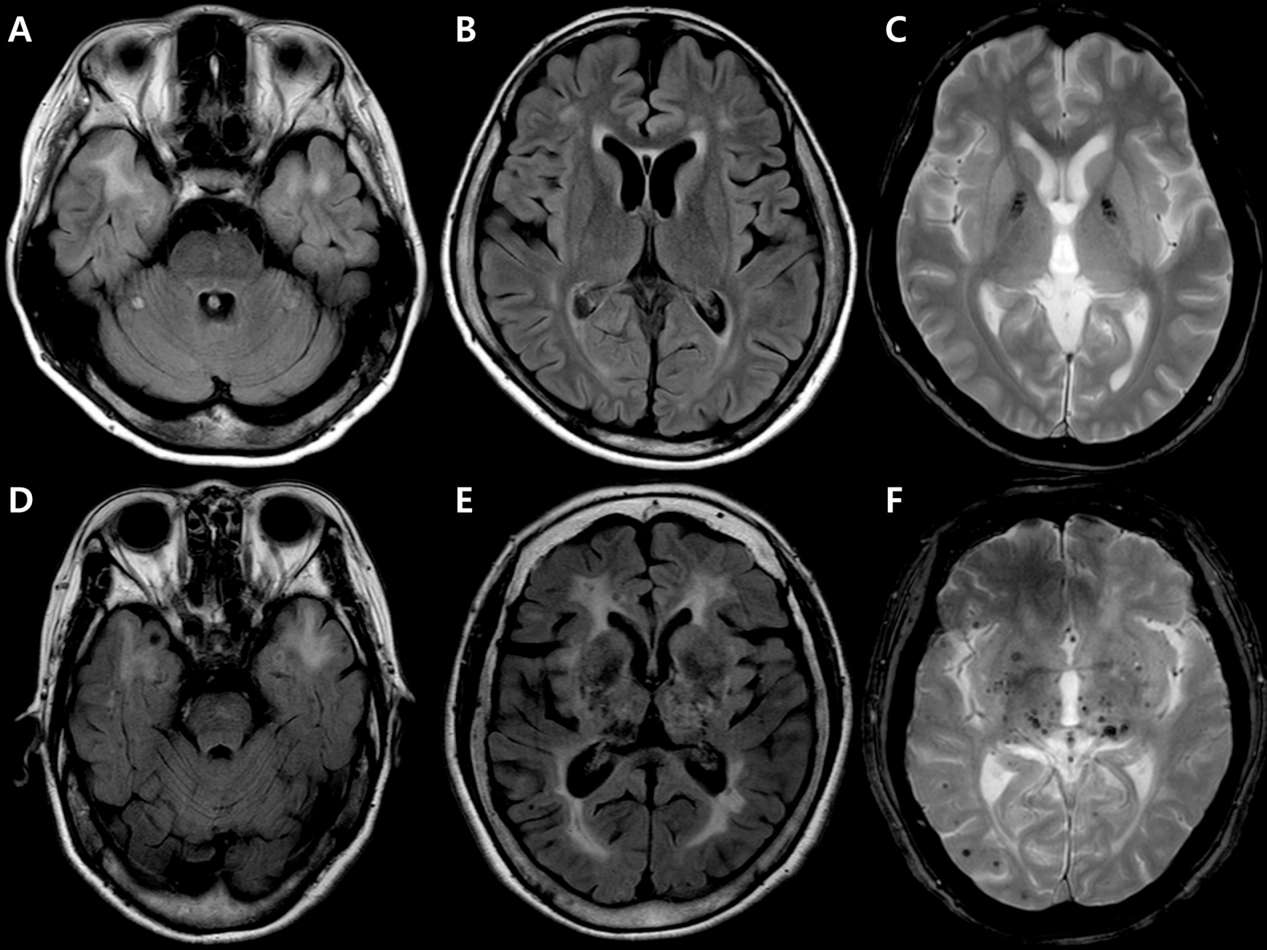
Representative MRI of patients with DM1 or CADASIL. Axial fluid attenuation inversion recovery (FLAIR) images showing bilateral anterior temporal hyperintensities in DM1 (A) and CADASIL (D). Axial FLAIR images showing more limited involvement of frontal, parieto-occipital, external capsule and basal ganglia regions in DM1 (B) compared with CADASIL (E). Axial gradient-echo T2*-weighted (GRE) images showing absence of microbleeds in DM1 (C) compared with multiple microbleeds observed in CADASIL (F).
